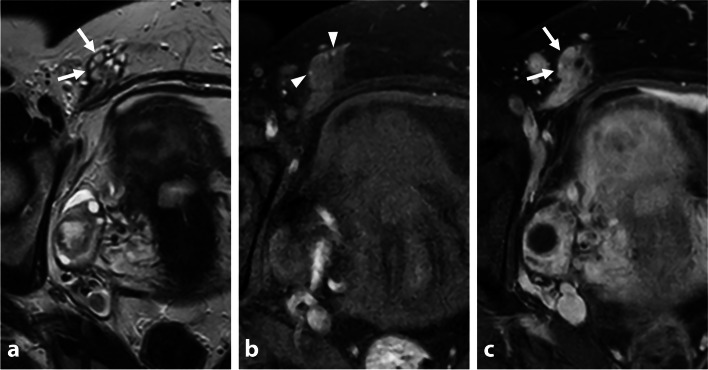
Right round ligament varicosity in the inguinal canal in a 33-year-old woman. a Axial T2-W MR images show pseudocystic changes of the right round ligament in its inguinal course (arrows). Thickening appears regular, without any fluid around the ligament. b Axial fat-suppressed T1-W MR image reveals a few T1-hyperintense foci (arrowheads) within the right round ligament, due to an “entry slice phenomenon artifact”. c Axial T1-weighted fat-suppressed contrast-enhanced MR image shows homogeneous enhancement of tubular veins around the right round ligament (arrows)
